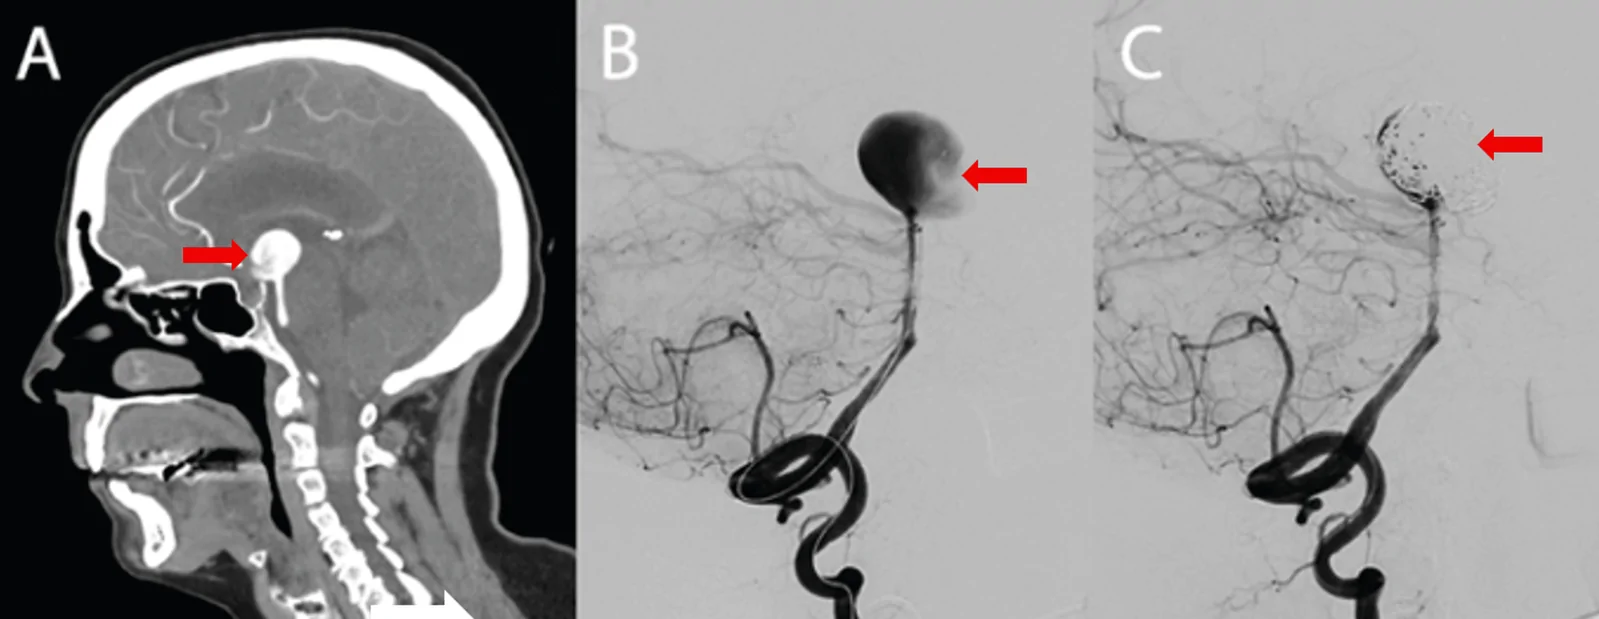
(A) CTA sagittal view showing large aneurysm of the tip of the basilar artery; (B) DSA showing large aneurysm of the tip of the basilar artery with mild contrast extravasation; (C) DSA showing basilar artery tip aneurysm status post endovascular coiling CTA: computed tomography angiography; DSA: digital subtraction angiography
